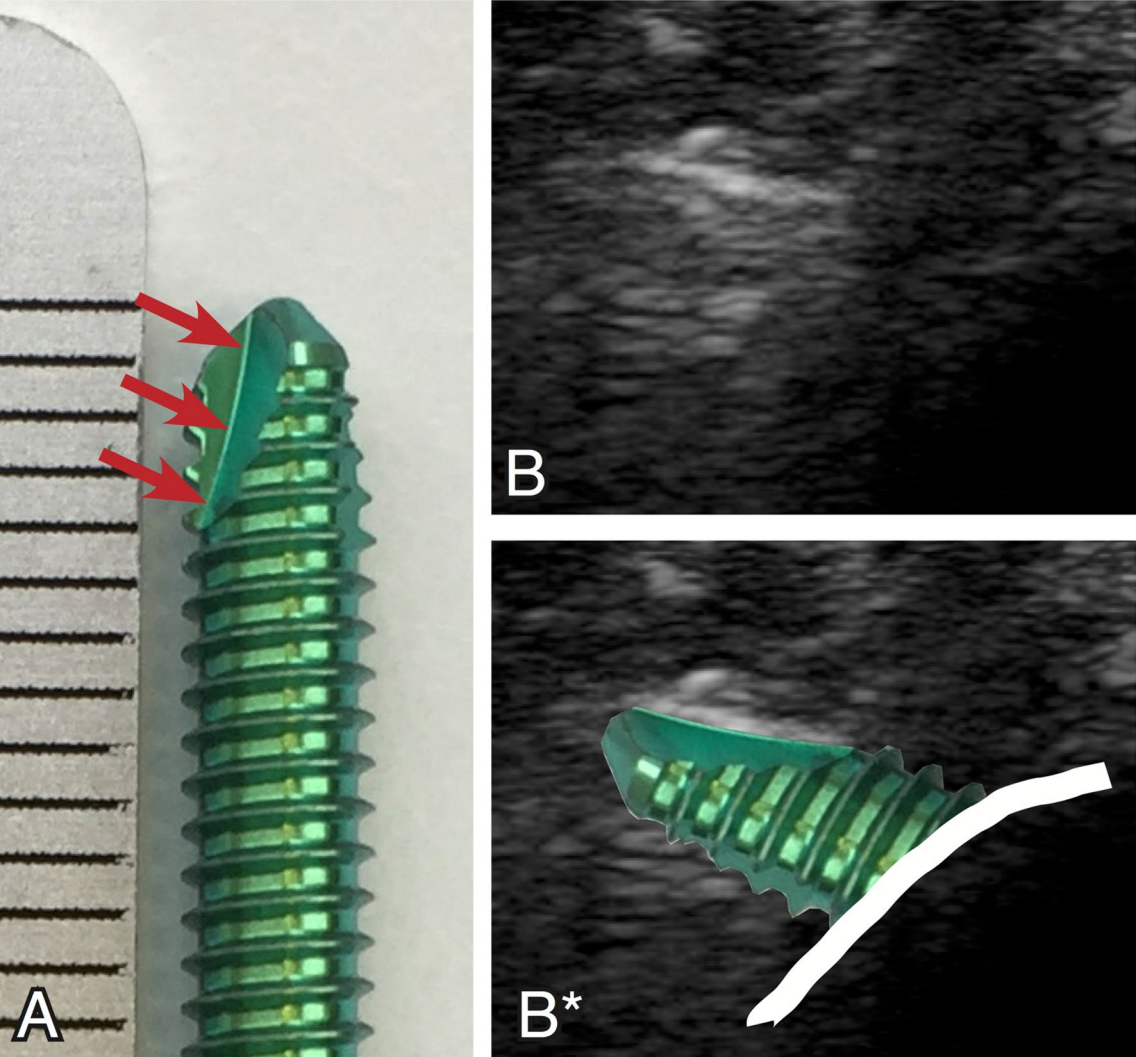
Additional ultrasound aspect of self-tapping screws. A: Self-tapping screw with a gap (red arrows) in the screw tip, which creates the cutting edge of the self-tapping screw. B: Orientation of the ultrasound plane almost rectangular to the gap in the screw tip causes absence of the characteristic thread-reflections. B* same image with the photo of the screw superimposed (color figure online)
